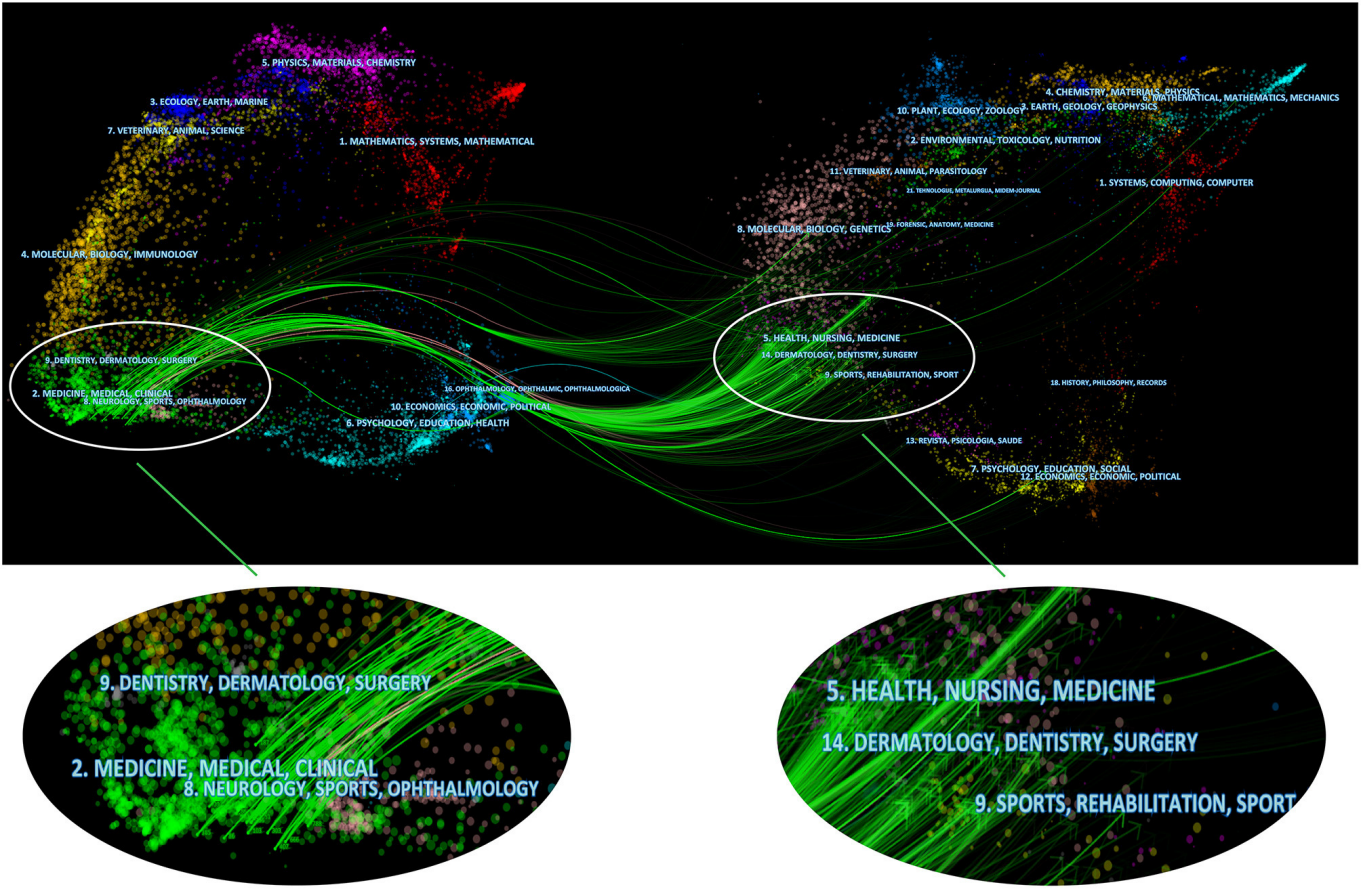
Related fields of the use of ultrasound in emergency medicine. The left side represents the fields of articles included in the study, and the right side represents the fields of references of articles.
